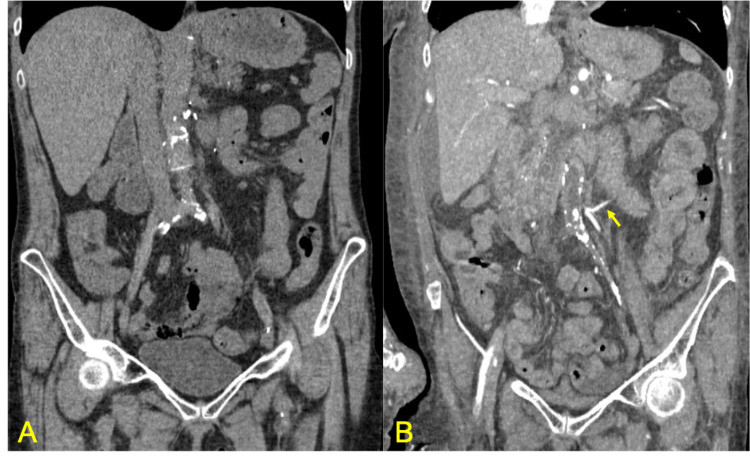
CT vs. CTA of abdomen and pelvis A: non-contrast CT of the abdomen and pelvis showed stable surgical changes and limited view of the vasculature. B: CT angiography (CTA) of the patient's abdomen and pelvis showed complete occlusion of abdominal aorta aneurysm graft and poor vasculature. A collateral vessel called the arc of Riolan (arrow) is shown, which is formed between the proximal superior mesenteric artery and the inferior mesenteric artery in the setting of severe vascular occlusion [5] CT: computed tomography
